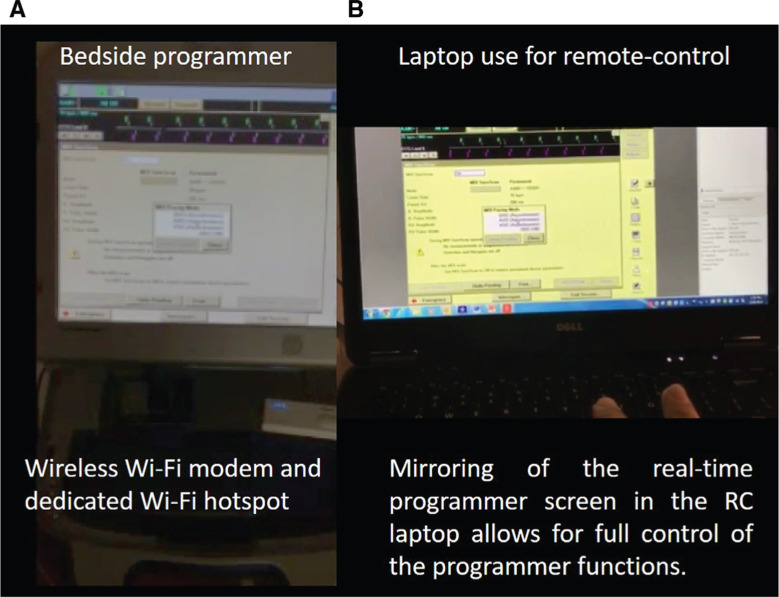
“Programmer” for the remote management of MRI-conditional cardiac devices. A: This computer had a connectivity modem card with options of intranet cable connection or a dedicated Wi-Fi cellular hotspot. The session was initiated by a bedside technician. B: Once informed that the patient was ready, the remote operator logged onto the session to access the RC functions of the programmer.
